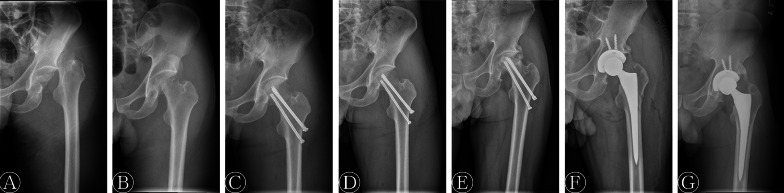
A 48-year-old man with a left Pipkin type III femoral head fracture. A Radiograph after injury. B Radiograph after attempted closed reduction of posterior hip dislocation. C Radiograph after open reduction and internal fixation. D Radiograph at 3 months postoperatively showing bony union. E Radiograph at 36 months postoperatively showing osteonecrosis of the femoral head and heterotopic ossification. F Radiograph after total hip arthroplasty. G Radiograph at final follow-up
